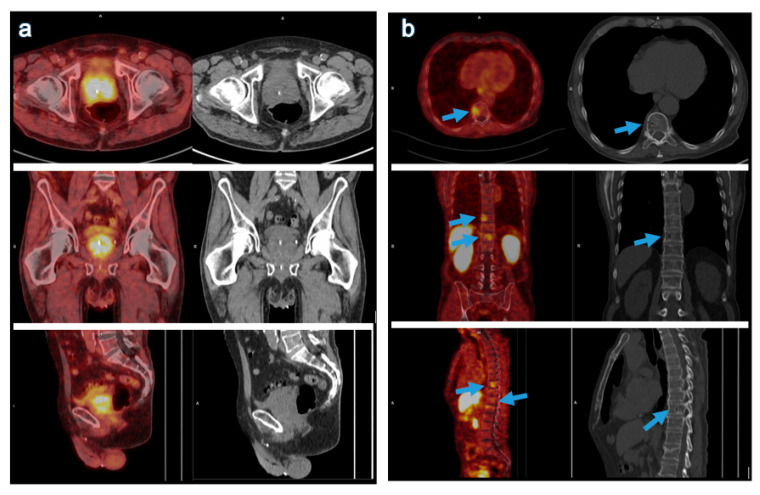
Primary staging of a treatment-naïve, 75-year-old patient with PCa which has metastasized to the bone, using [^64^Cu]-DOTAGA-PSMA PET–CT. The PSA value at the time of PET–CT was 47 µg/L. (a) The PSMA-positive primary tumor (SUVmax apical: 9.5; left base: 8.0). (b) The PSMA-positive bone metastases in T10 and T12 (respective SUVmax: 5.3 and 4.6); the arrow in the correlating low-dose CT images point to the metastasis in T10.
